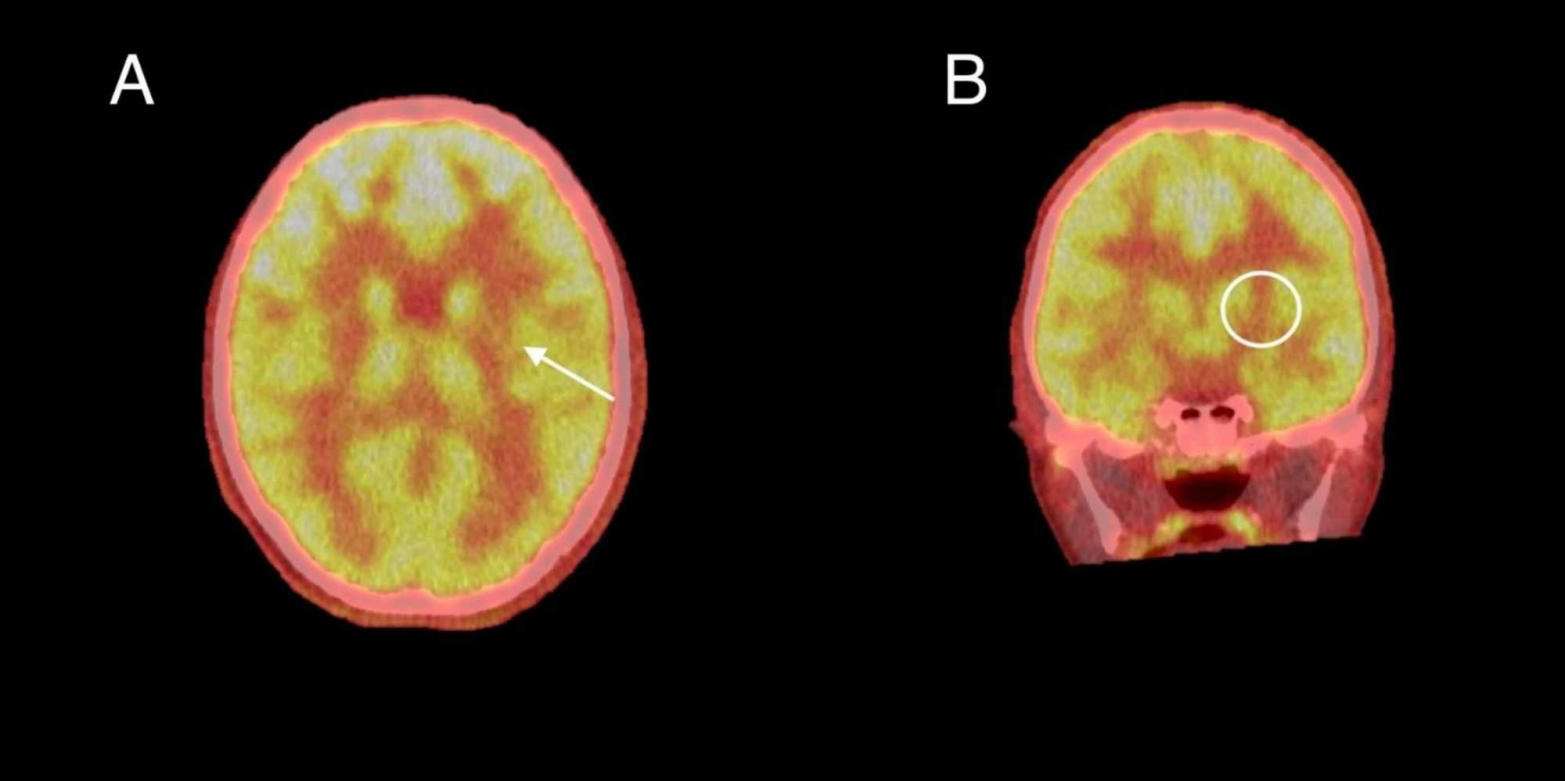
Axial coronal PET/CT fused image of the brain (Figure 2A) demonstrate asymmetric diminished activity within the left basal ganglia, as compared to the right (white arrow). This relative decreased activity is further demonstrated on the coronal PET/CT fused image of the brain (white circle in Figure 2B). PET/CT: positron emission tomography/computed tomography
